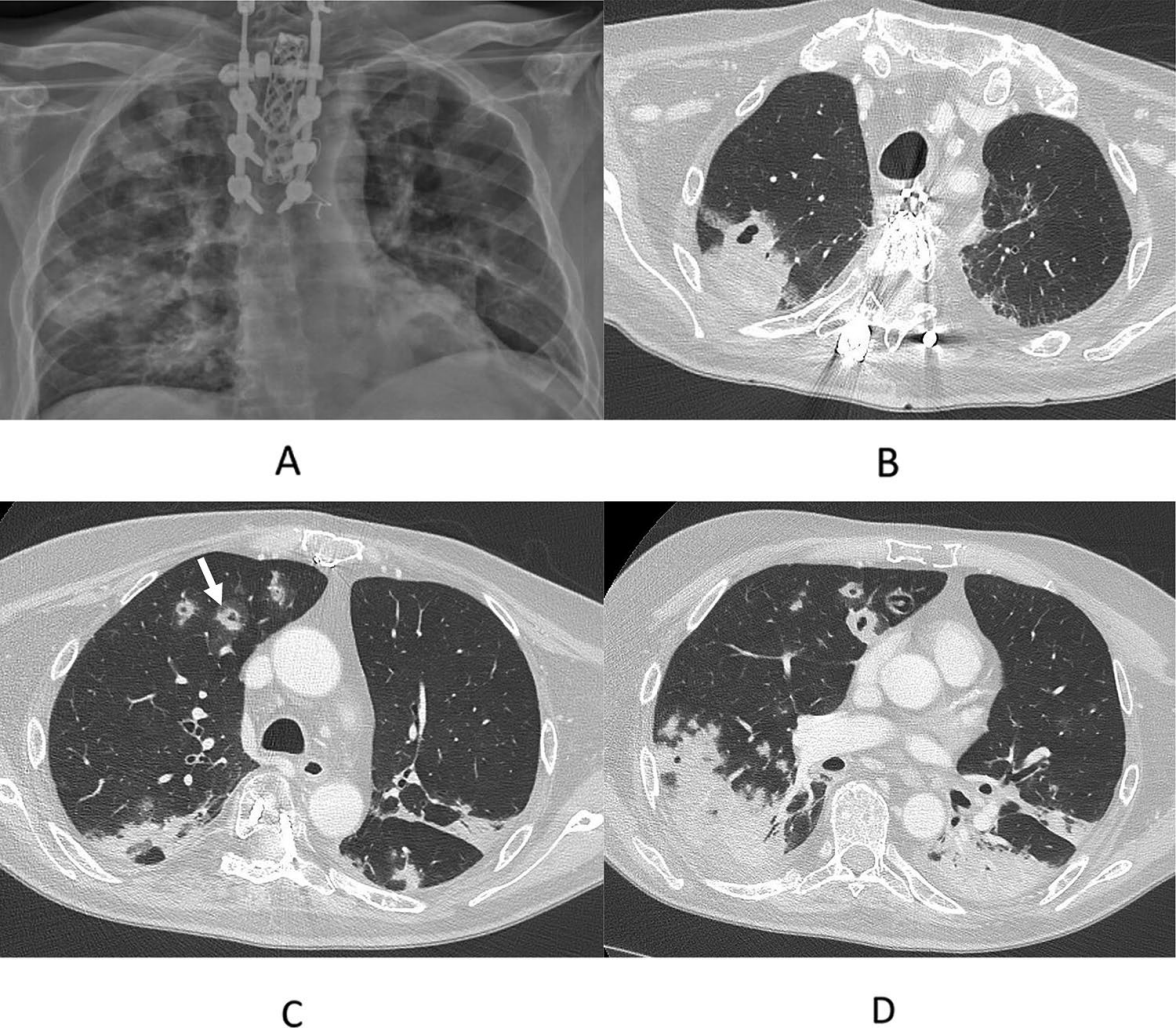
Radiological images of case 1. A Chest radiography. Bilateral nodular consolidations. B–D CT chest scan. Cavitated nodules and mass. Halo sign (white arrow)
